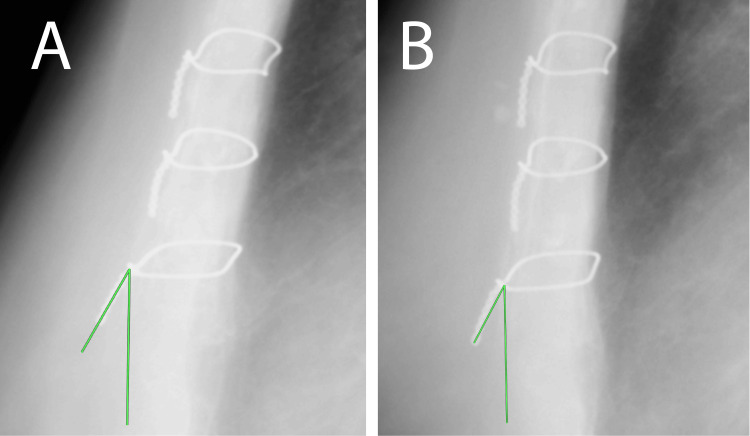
Chest X-p after two and six years (A) Two years post-procedure. The angle between the vertical line is 27.2° (green line). (B) Six years following the procedure. The angle between the vertical line is 28.8° (green line). Chest X-p: chest X-ray photograph
